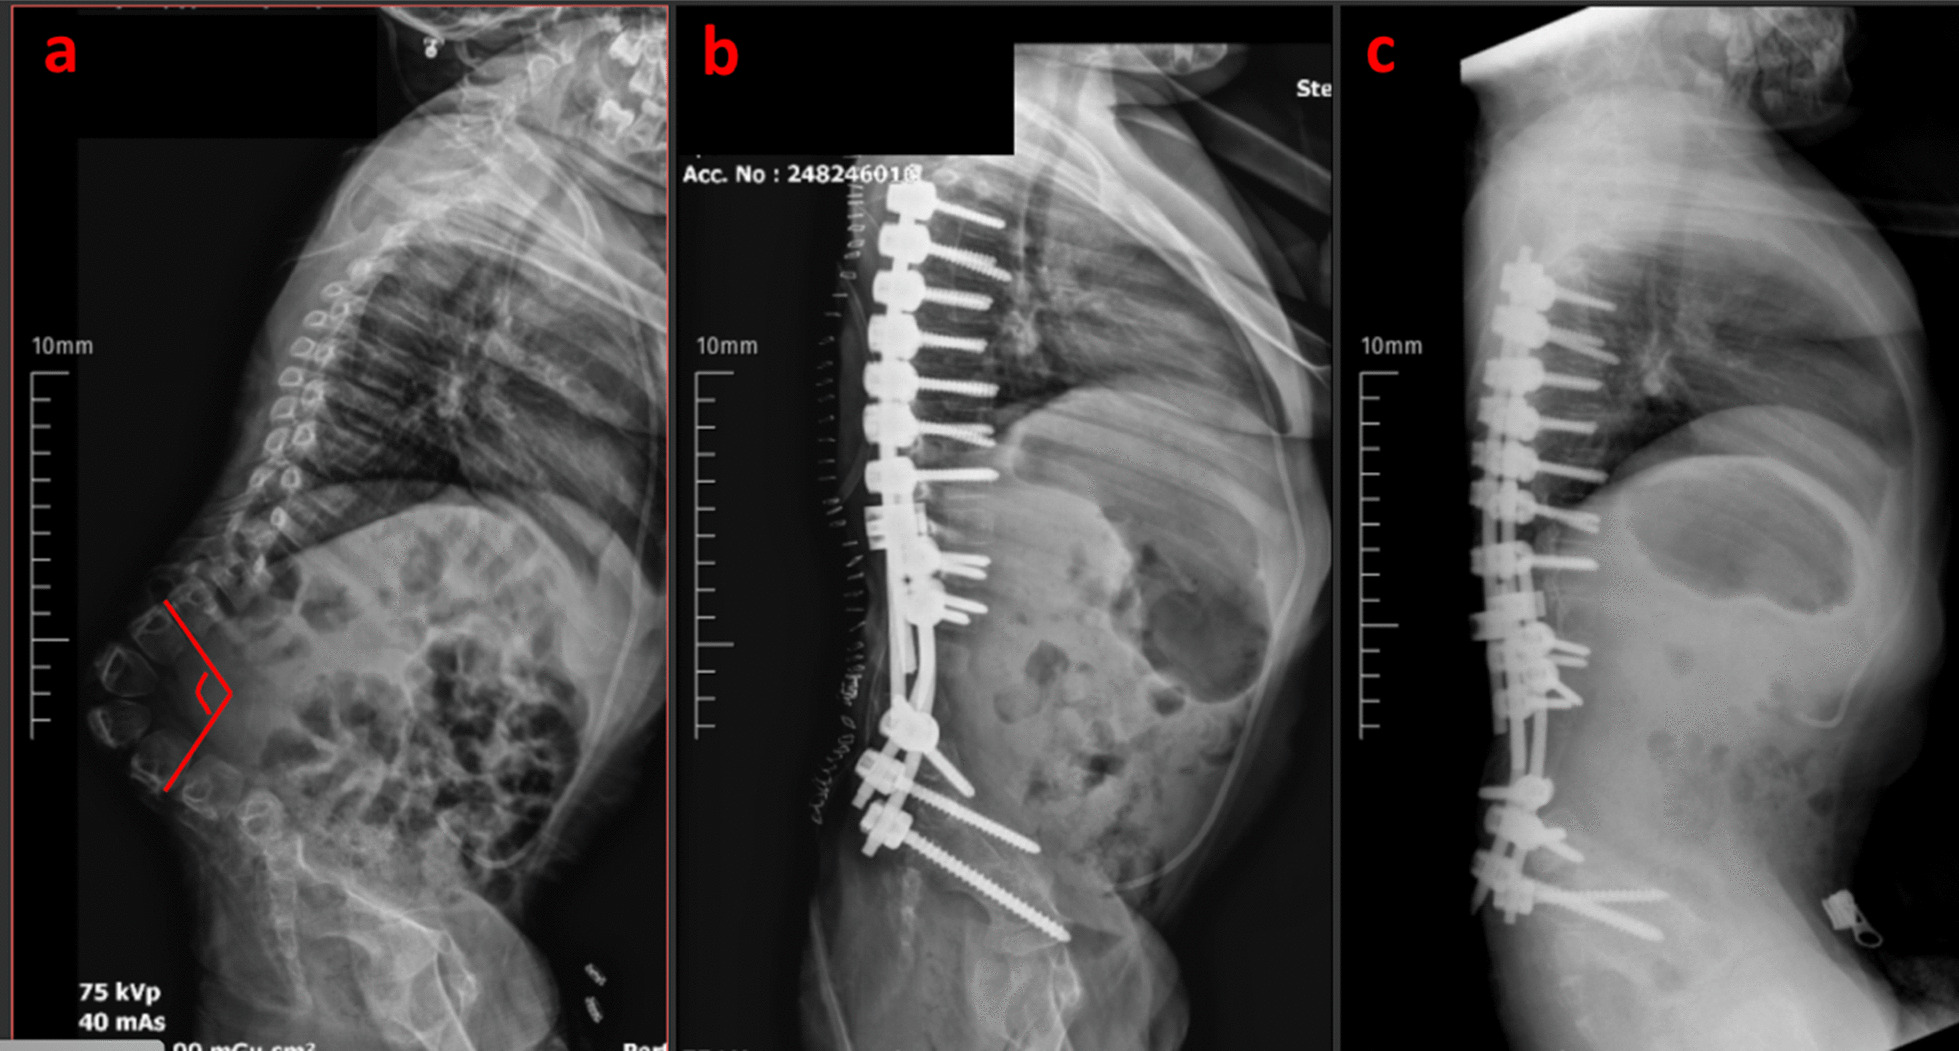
Preoperative and postoperative altered kyphosis angle. A. Preoperative kyphosis angle 118°. B. Early postop kyphosis angle – 1 °. C. Radiograph at last follow-up
